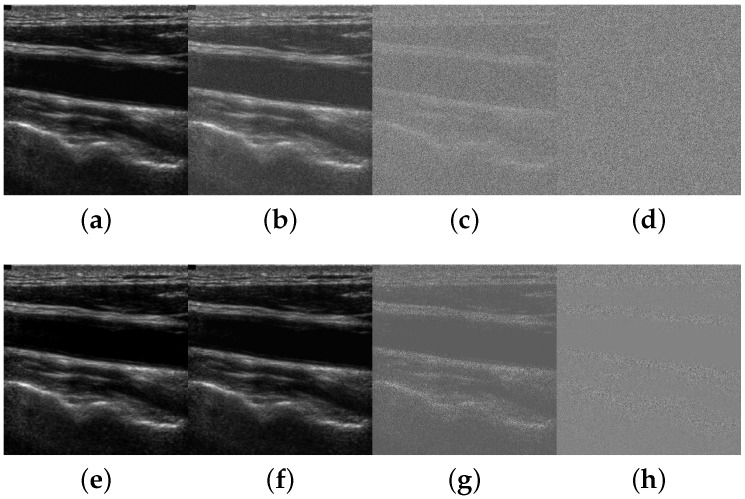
Examples of carotid ultrasound images with different levels of Gaussian (first row) and speckle (second row) noise. (a) 30 dB; (b) 10 dB; (c) -10 dB; (d) -30 dB; (e) 30 dB; (f) 10 dB; (g) -10 dB; (h) -30 dB.
